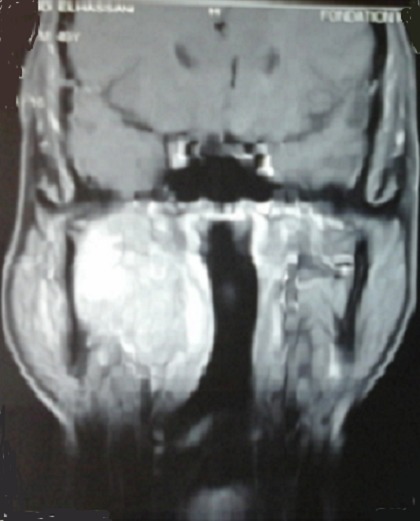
MRI appearance before radiotherapy.
